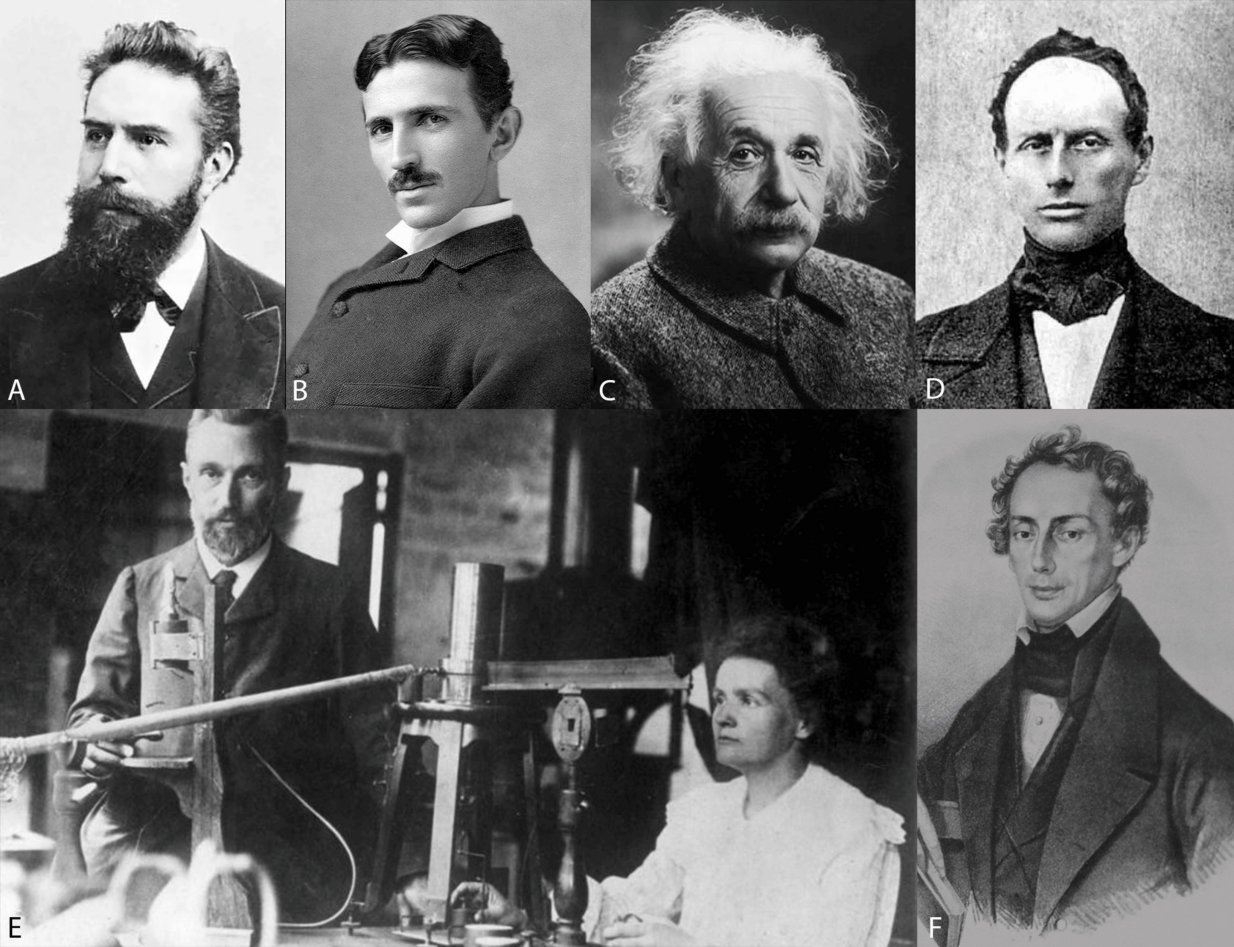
Original images from Wikipedia A. Wlihelm Conrad Roentgen photograph, B. Nikola Tesla photograph, C. Albert Einstein photograph, D. Christian Doppler photograph, E. Pierre and Marie Currie photograph, F. Chrisitan Doppler vintage sketch. (All images are in the public domain, obtained from Wikipedia)
